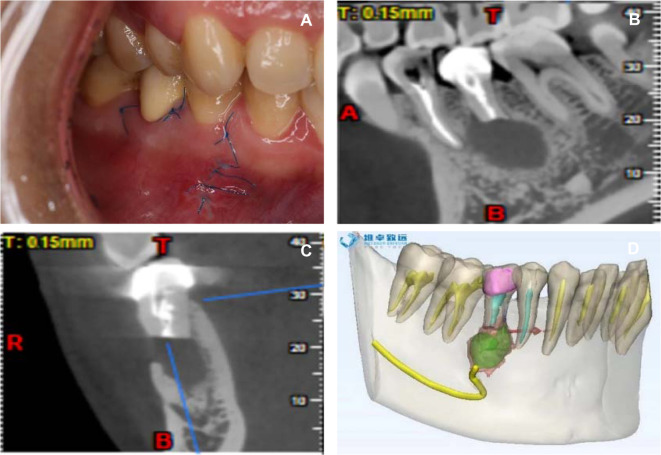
The CBCT images were reviewed. (A) One week postoperative wound condition (B) Complete resection of apical lesions (C) Distance from the inferior alveolar nerve canal in the lesion area after surgical resection (D) Resection range covers the extent of the lesion.
